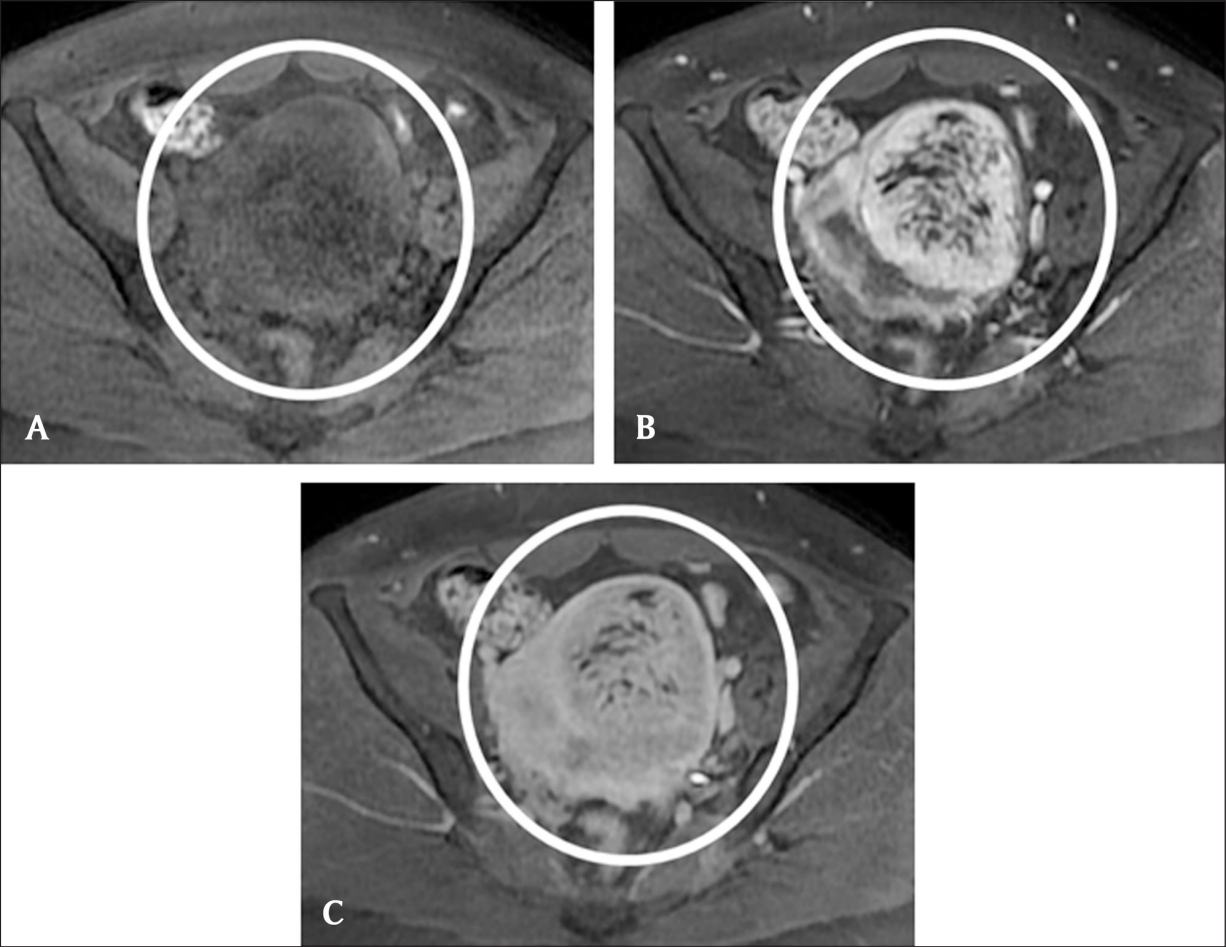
(A) Unenhanced axial T1-weighted MR image: the hypointense uterine lesion in the center of The circle. (B) Enhanced axial T1-weighted MR image: the arterial phase shows avid captation. (C) Enhanced axial T1-weighted MR image: the light tumoral washout on the delayed phase.
